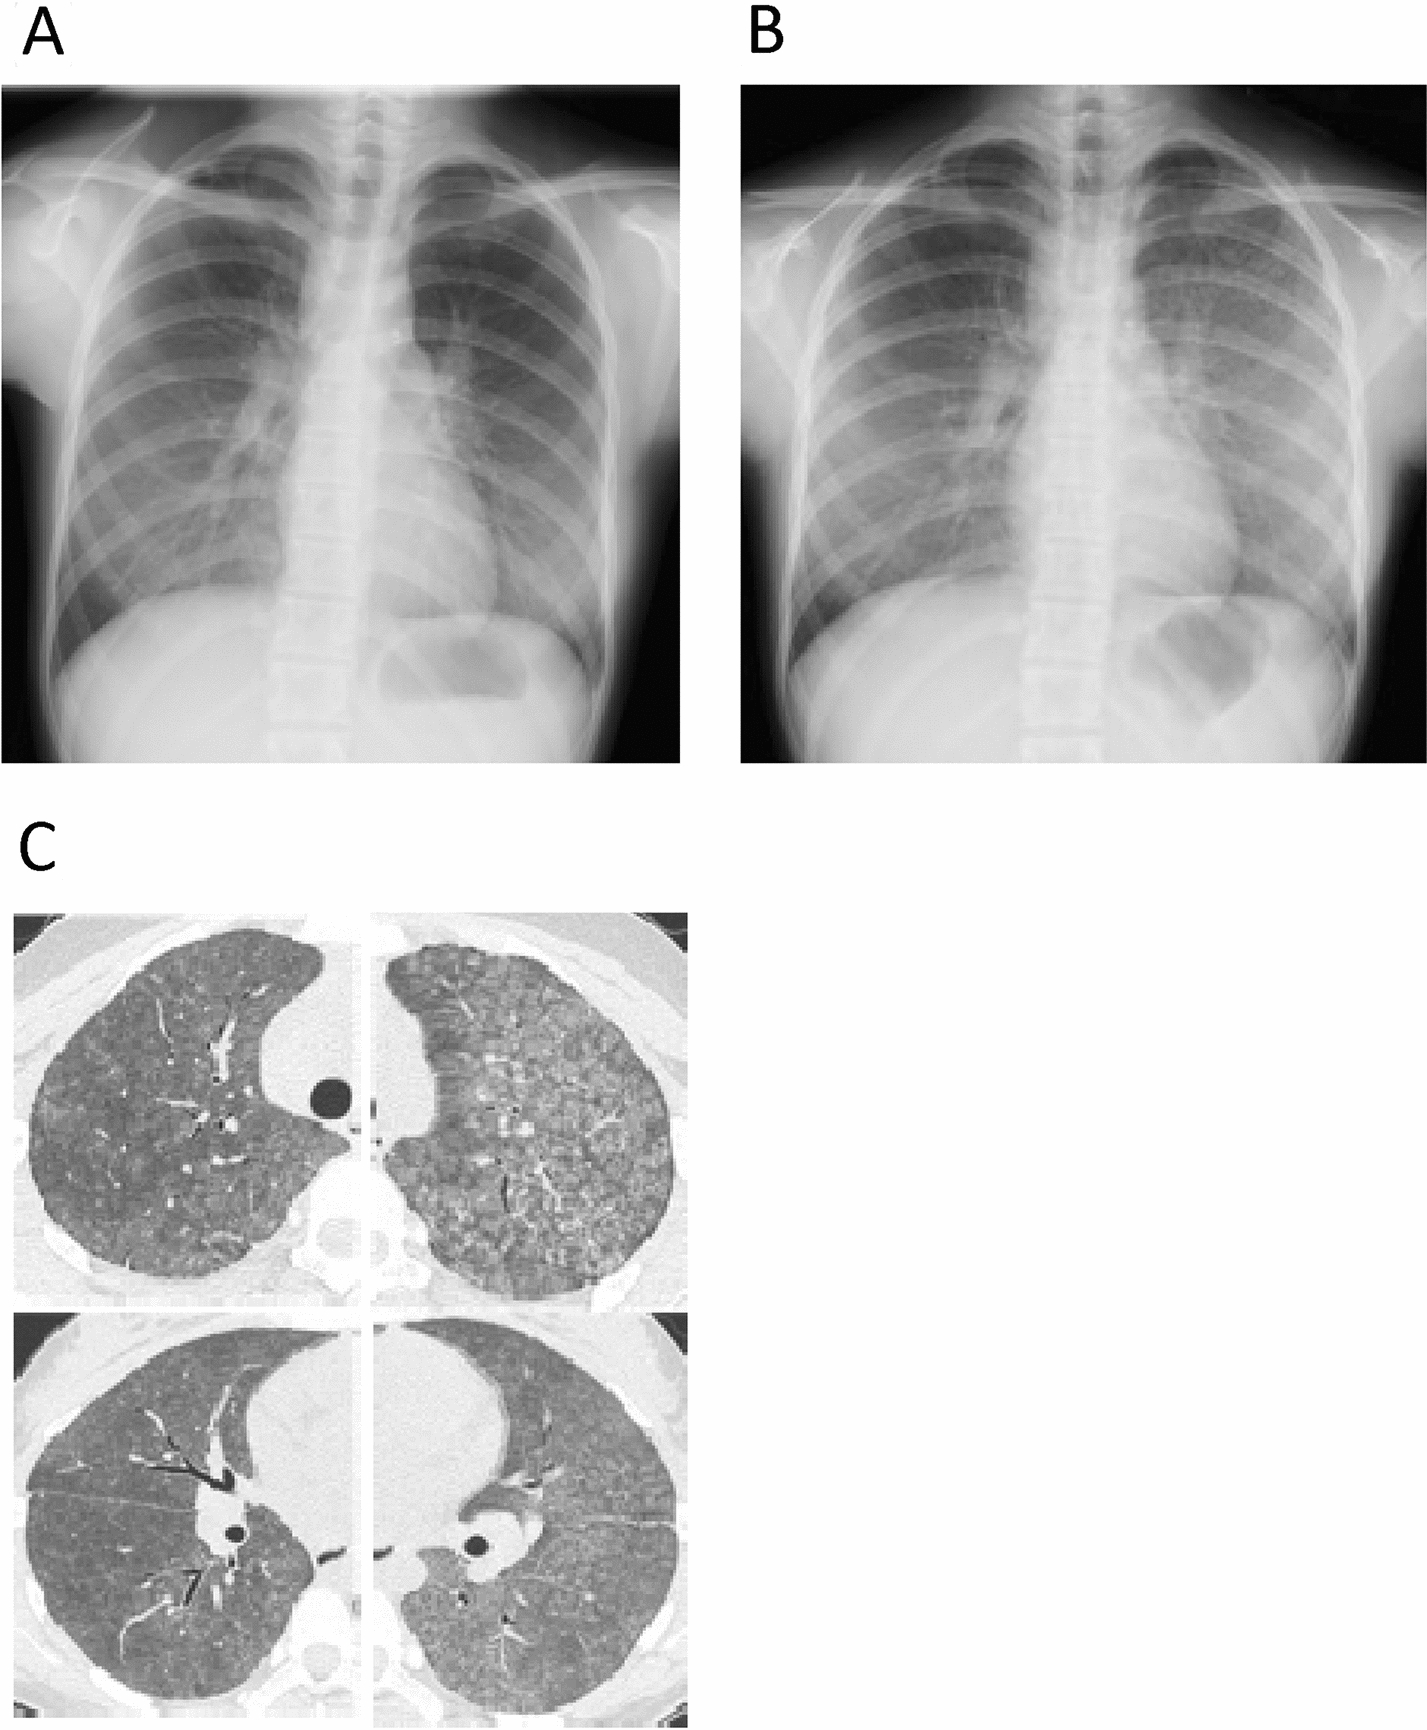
Chest X-ray images before and after tocilizumab administration and chest CT image after tocilizumab administration. Chest X-ray images before (A) and 5 days after (B) tocilizumab administration and chest CT image 5 days after tocilizumab administration (C)
